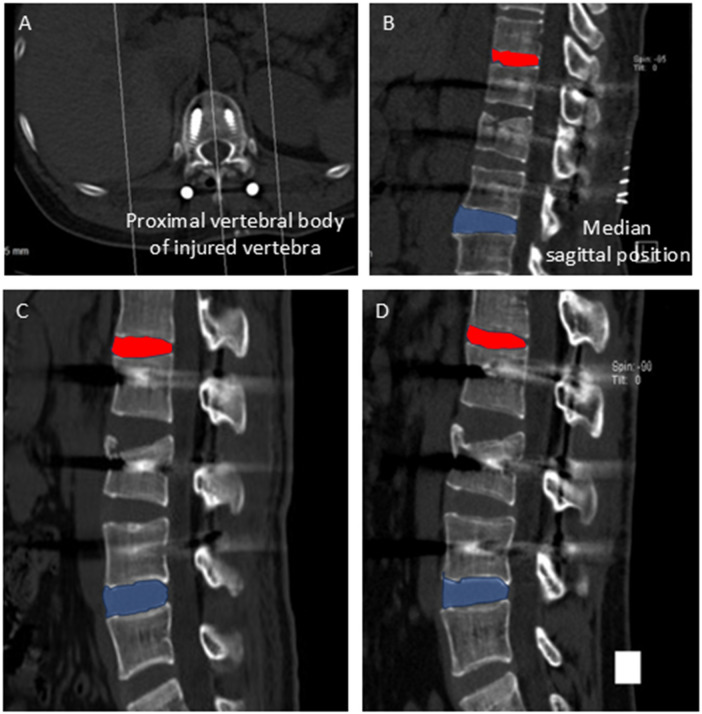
Radiographic change of intervertebral disc degeneration in adjacent segments after short segment fixation. (A,B) Selection of the measurement layer of intervertebral disc area: median sagittal position of the proximal vertebral body of the injured vertebra. Red area represents proximal intervertebral disc, and the blue area represents distal intervertebral disc. (C,D) Case 3, male, 25 years old, L3 fracture. A CT scan executed one week post-op (C) and fifteen months post-op (D) clearly shows reduction of sagittal cross-sectional area of intervertebral disc in adjacent segments after short segment fixation.
